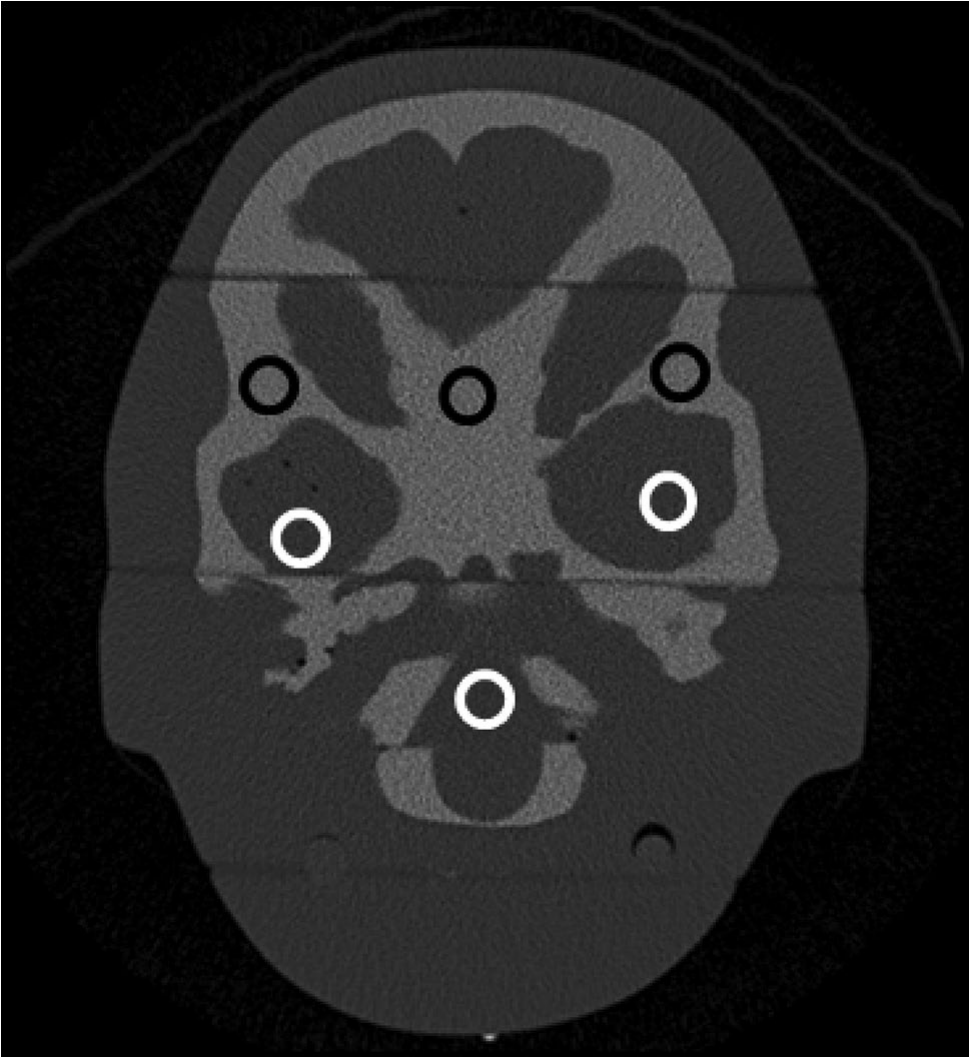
Example image (Revolution CT) with the regions of interest (*black circles* = bone, *white circles* = soft tissue) to determine the signal difference to noise ratio
